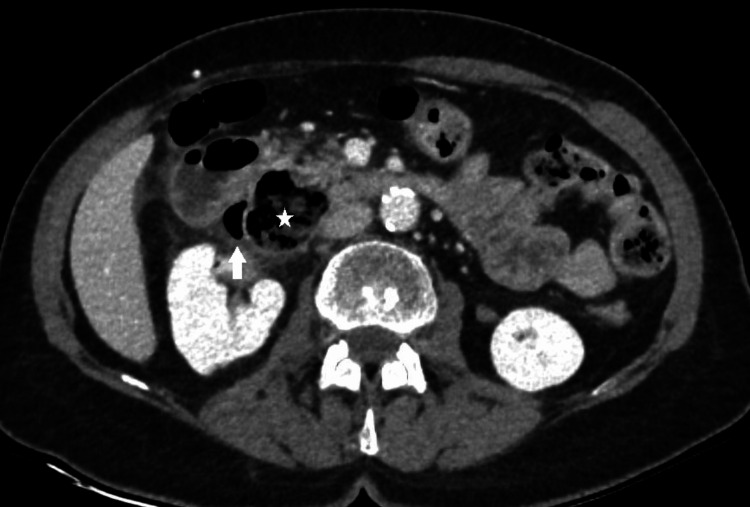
Initial CT of the abdomen and pelvis - axial image. Duodenal diverticulum (white star) localized on the medial wall of the descending duodenum at the pancreatic side with peridiverticular and periduodenal "fat-stranding" and a small amount of extraluminal gas (white arrow) near the medial duodenal wall - CT signs of perforation.
